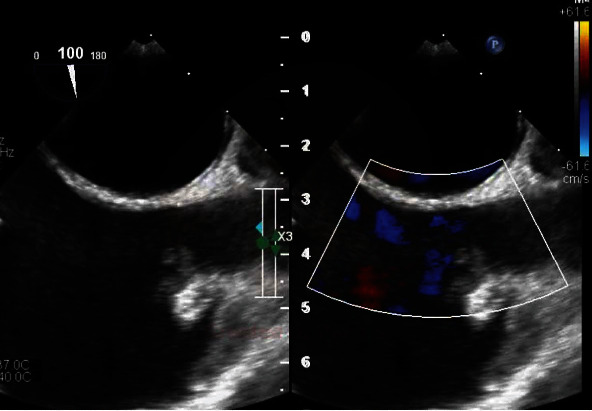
Transesophageal echocardiogram with color Doppler showing mobile thrombus at the junction of the SVC and right atrium.
